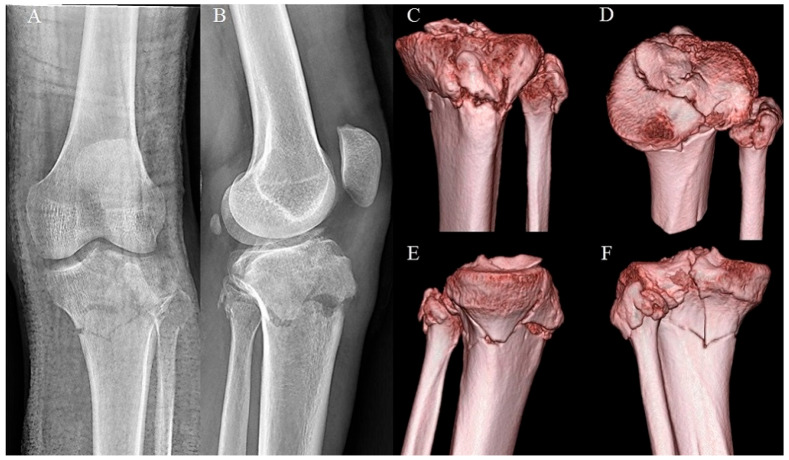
A 35-year-old male. (A,B) Preoperative anteroposterior and lateral plain X-ray shows a left proximal tibial fracture. (C–F) 3D CT shows bicondylar fracture including metaphyseal-diaphyseal discontinuity. This type is Schatzker type VI and high-energy fracture.
